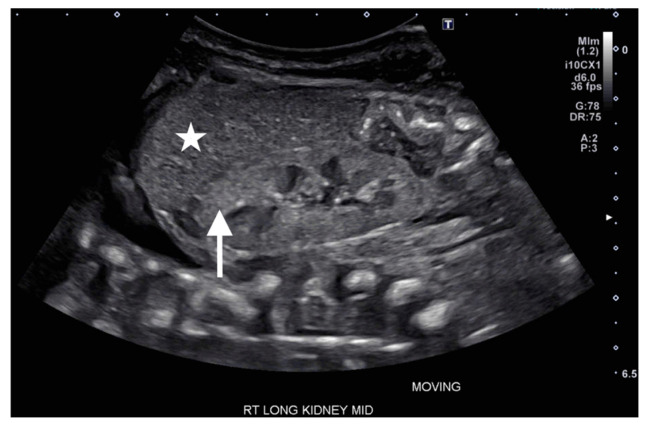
Ultrasound of right kidney on day of life 5 showing increased echogenicity (arrow) compared to that of the adjacent liver (asterisk).
